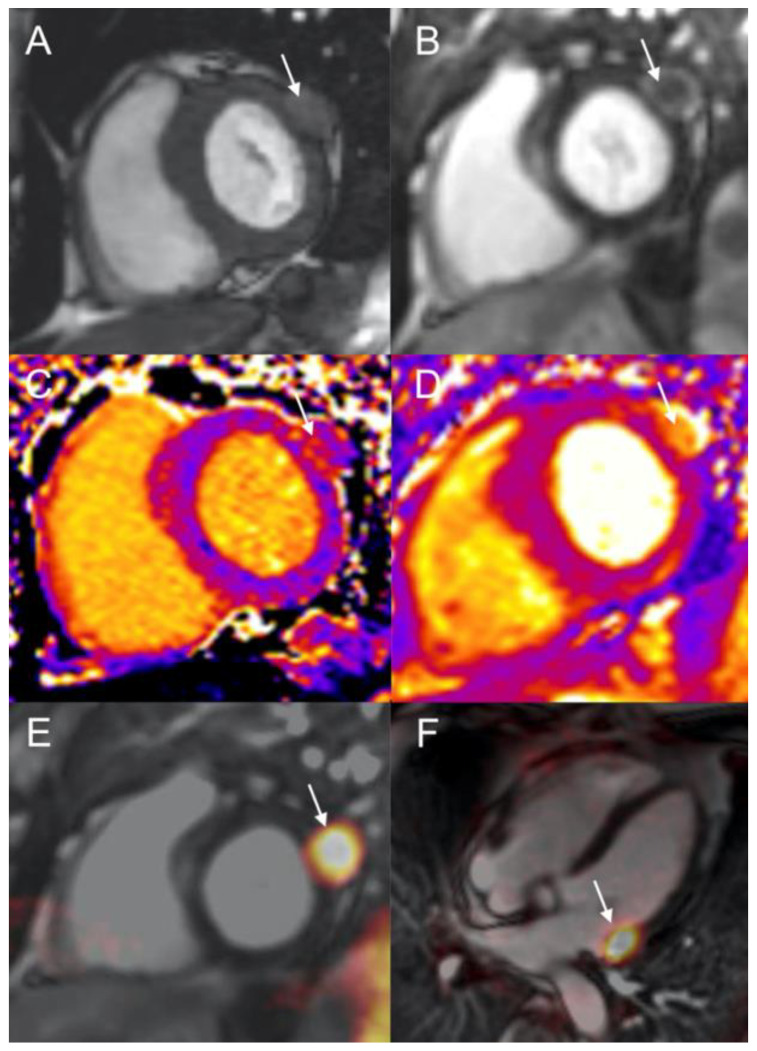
Patient 2. CMR showing a NEN metastasis of lateral basal LV wall (arrows) appearing as 25-mm nodule with intermediate intensity on SSFP T1-weighted images (A), with peripheral contrast-enhancement on phase sensitive inversion recovery sequence (PSIR) (B). T1- (C) and T2-mapping images (D) revealed an increased T1 (1172 ms), and T2 (81 ms). Fused ^68^Ga-DOTATOC PET/CMR: short axis (E) and 4-chamber view (F).
